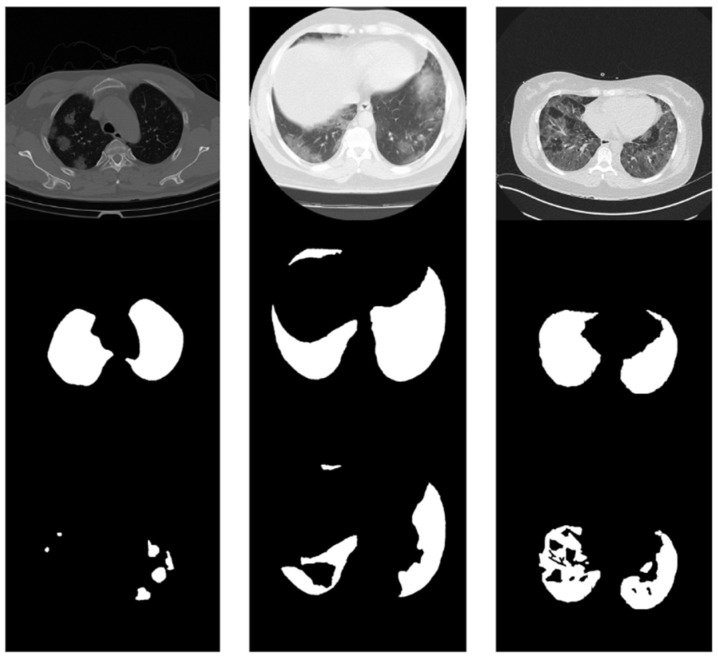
Examples of slices of CT scans after preprocessing. From top to bottom, a slice, a lung mask, and a COVID-19 mask.
